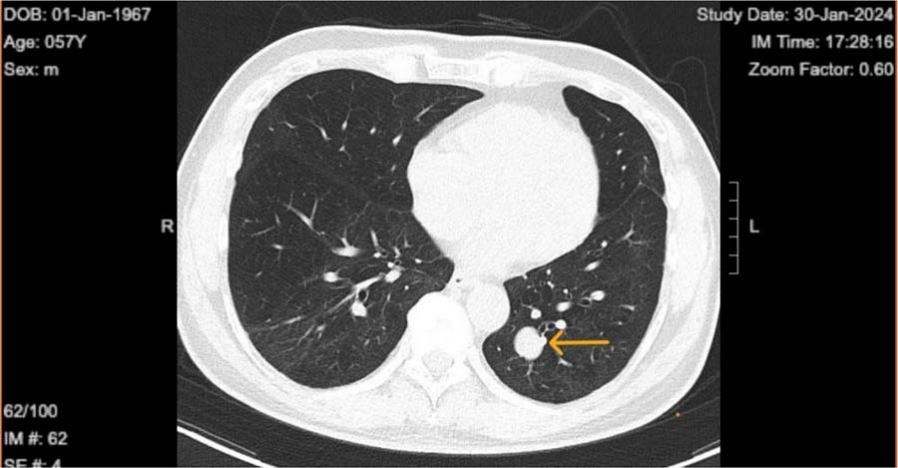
Chest computed tomography in 2024 shows well circumscribed solitary lung tumor of 1.9 × 1.6 cm in size.
